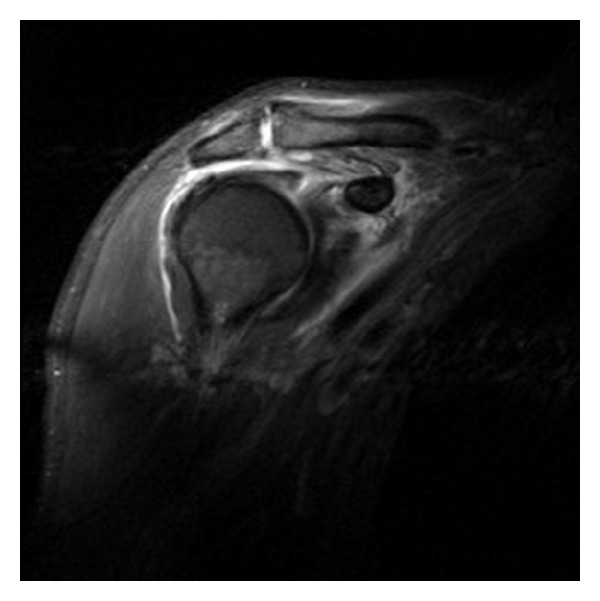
Coronal T2-weighted MRI scan showing collection in subacromial bursa.
